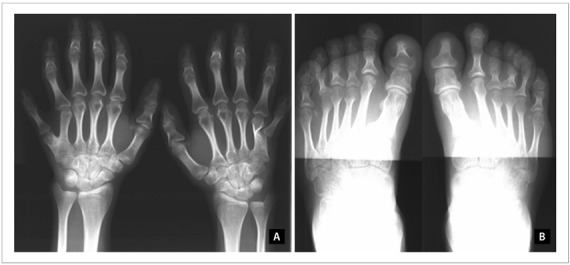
Radiographs on the patient’s hands and feet. Note especially the post-axial polydactyly, brachydactyly with short metacarpal and metatarsal bones and phalanges, and conical epiphyses (A and B).
